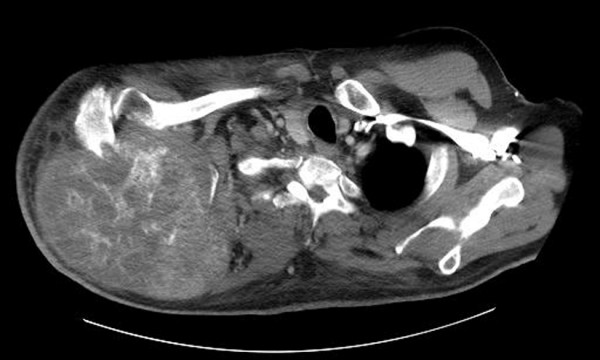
Preoperative CT scan demonstrating large scapular mass.
